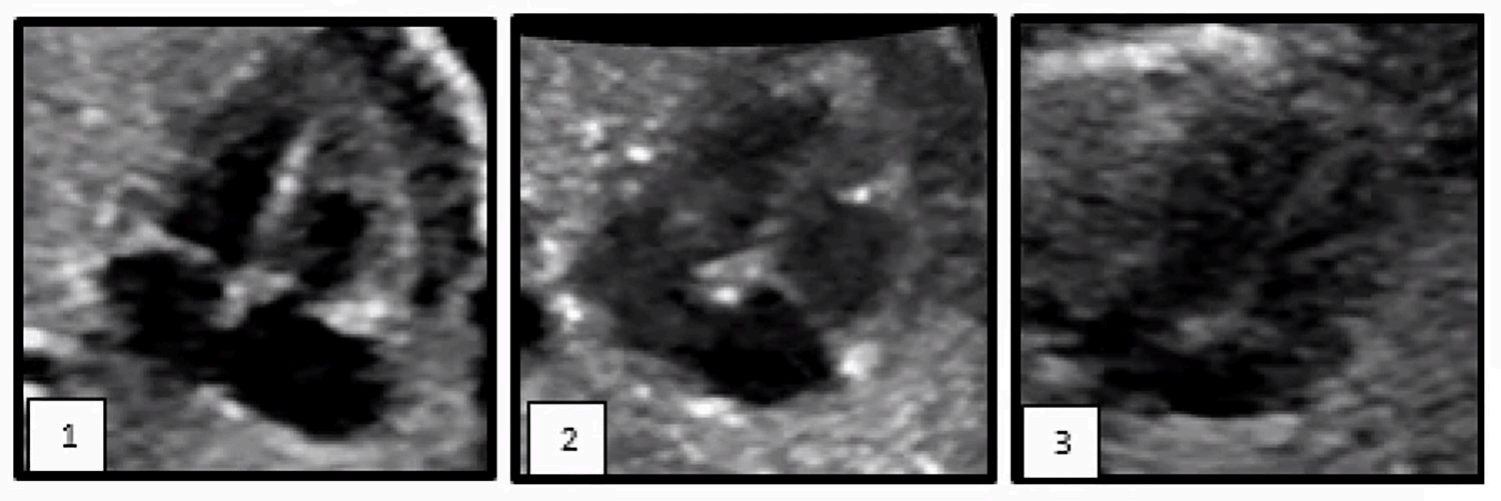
Evaluation of quality of four-chamber view ultrasound clips.
